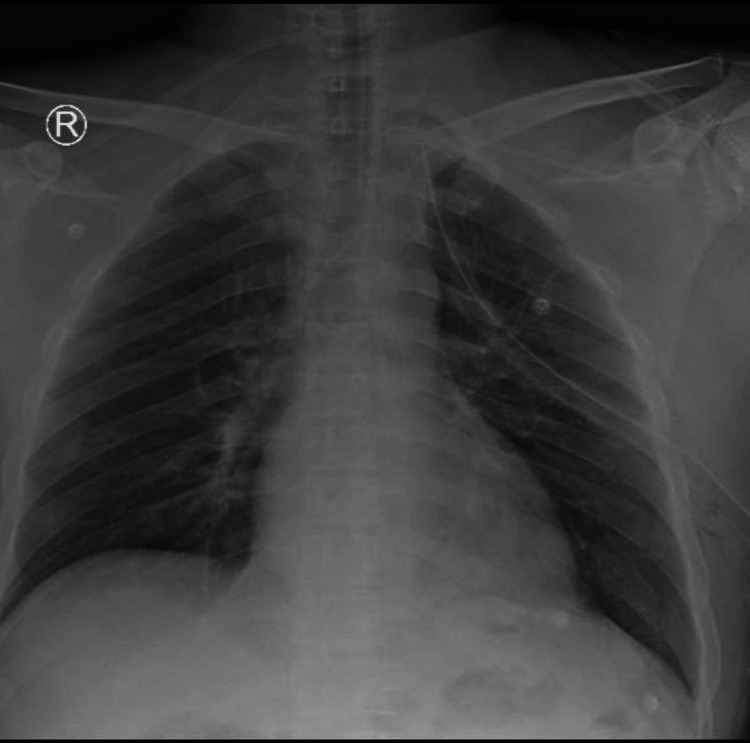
Chest X-ray after the operation with a chest tube in place.
